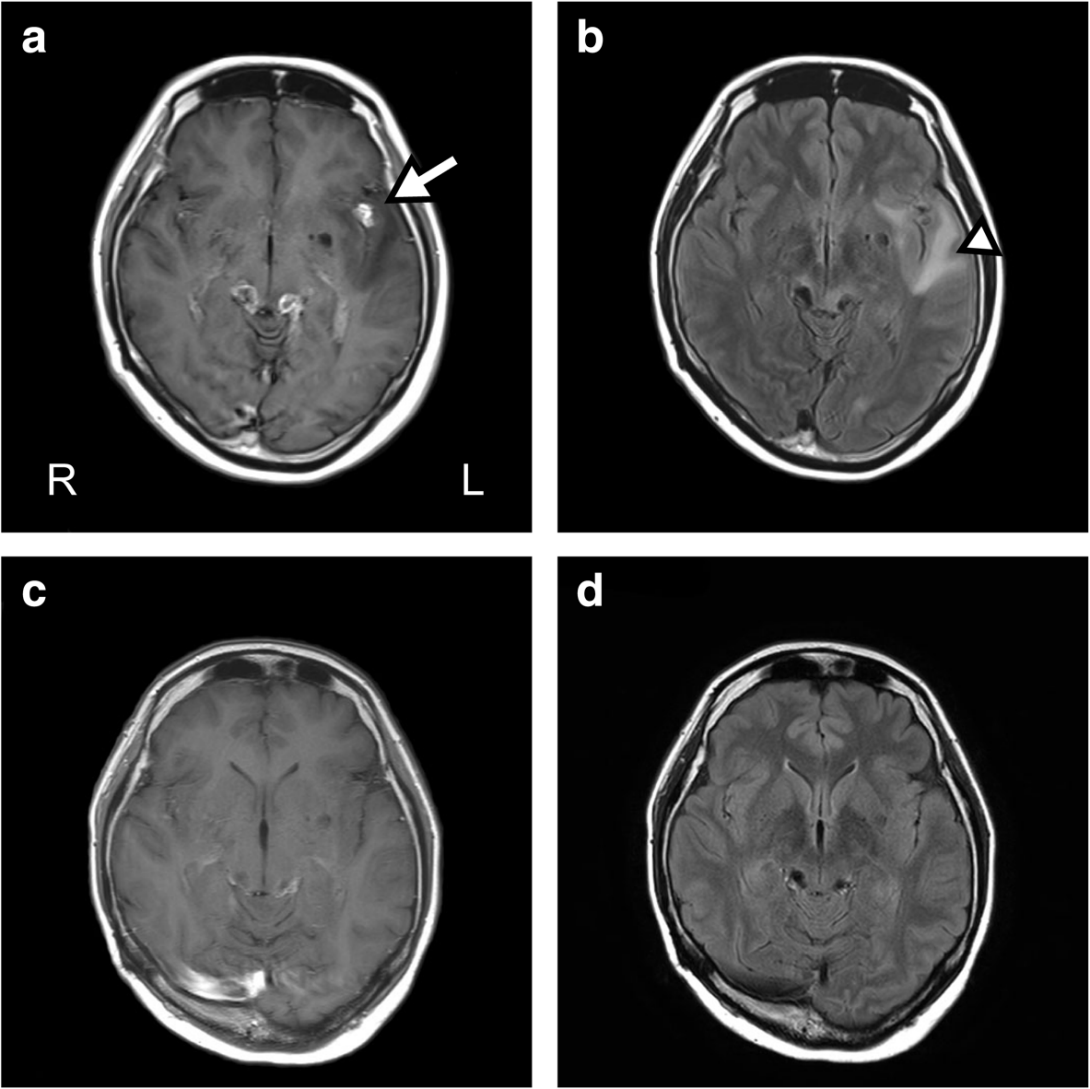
Temopral changes of the brain MRI after PR. a, Contrast-enhanced axial T1-weighted brain MRI revealed tuberculomas (arrow) with a diffuse hypointense area around the left sylvian fissure. b, Brain FLAIR imaging indicated a hyperintense area around the tuberculomas in the left syluvian fissure, which suggests edematous changes (arrowhead). c and d, After 3 months of the corticosteroid treatment, the tuberculomas disappeared on contrast-enhanced axial T1-weighted brain MRI, and edematous brain changes around the left sylvian fissure vanished in brain FLAIR imaging
